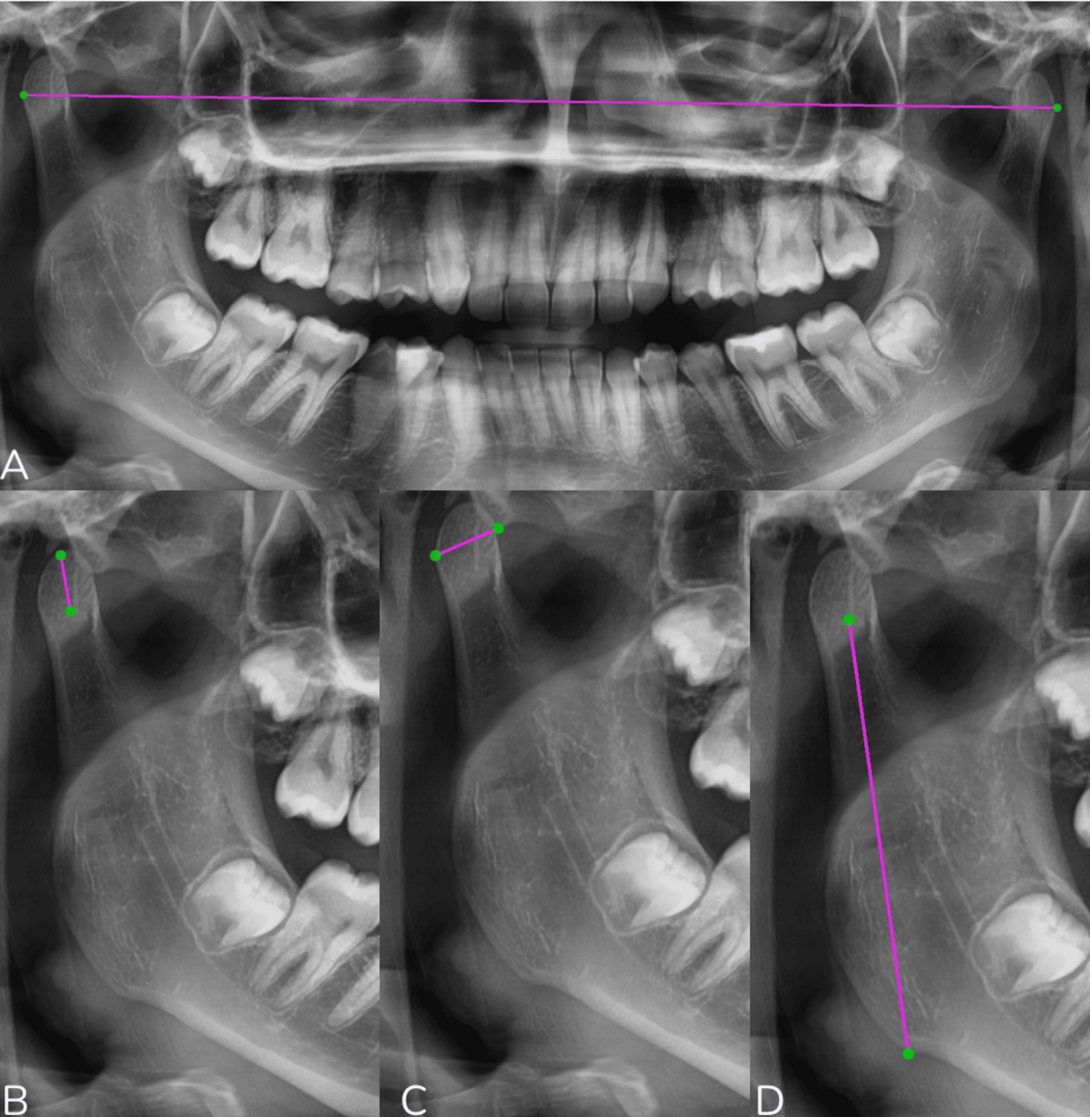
Panoramic radiographic measurements used in the study. (A) Measurement of intercondylar distance, defined as the linear distance between the most lateral points of the right and left mandibular condyles.
